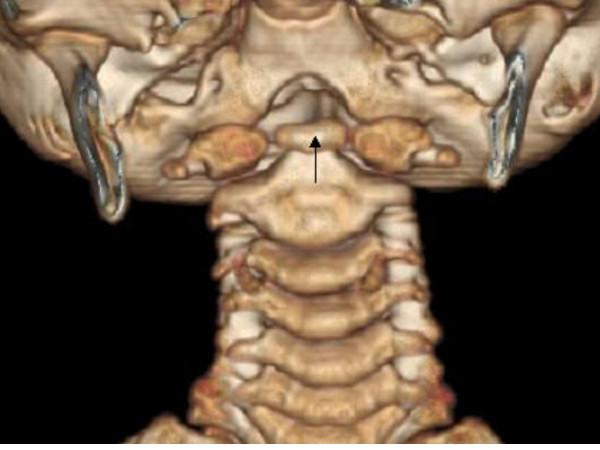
3D reconstruction CT scan ; Hypoplastic anterior arch of the atlas and the impacted os terminale of the odontoid (arrow) between the two halves of the maldeveloped anterior arch of the atlas-the os terminale usually fuses at 12 years of age-this can be confused with fracture.
